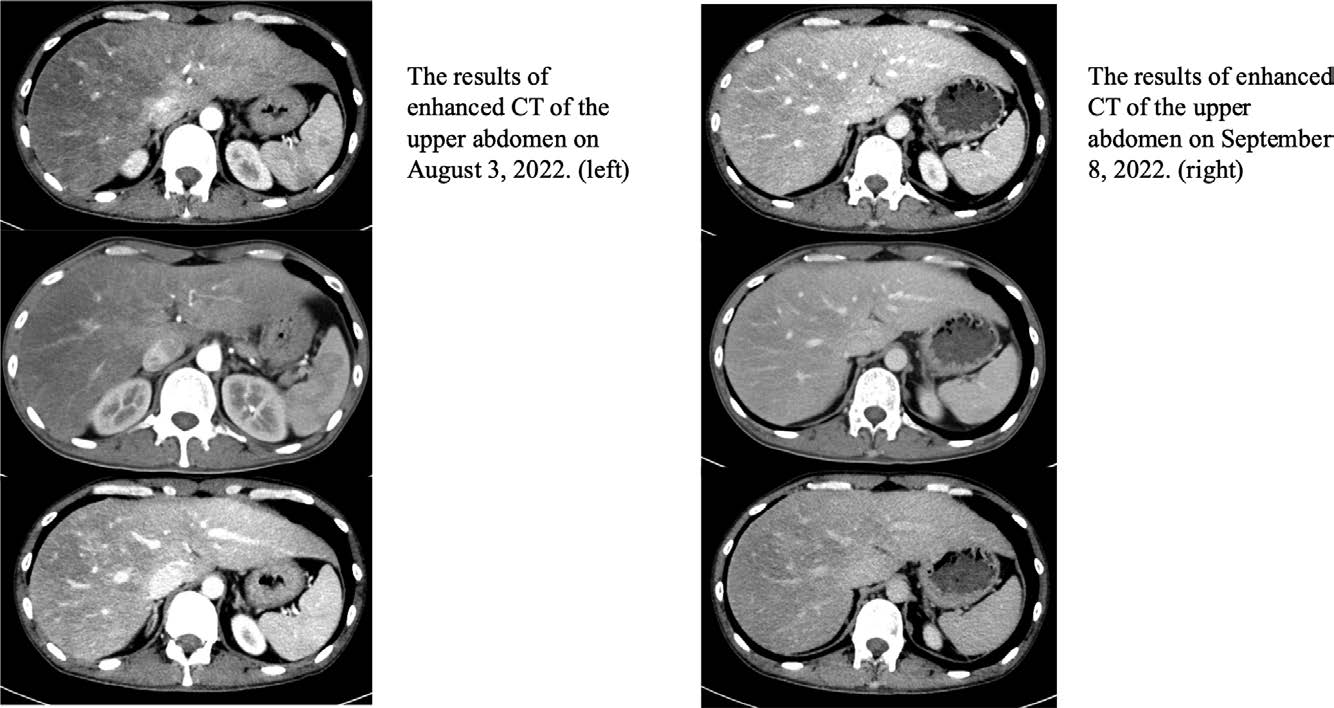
Enhanced CT scans of patients before and after treatment.
